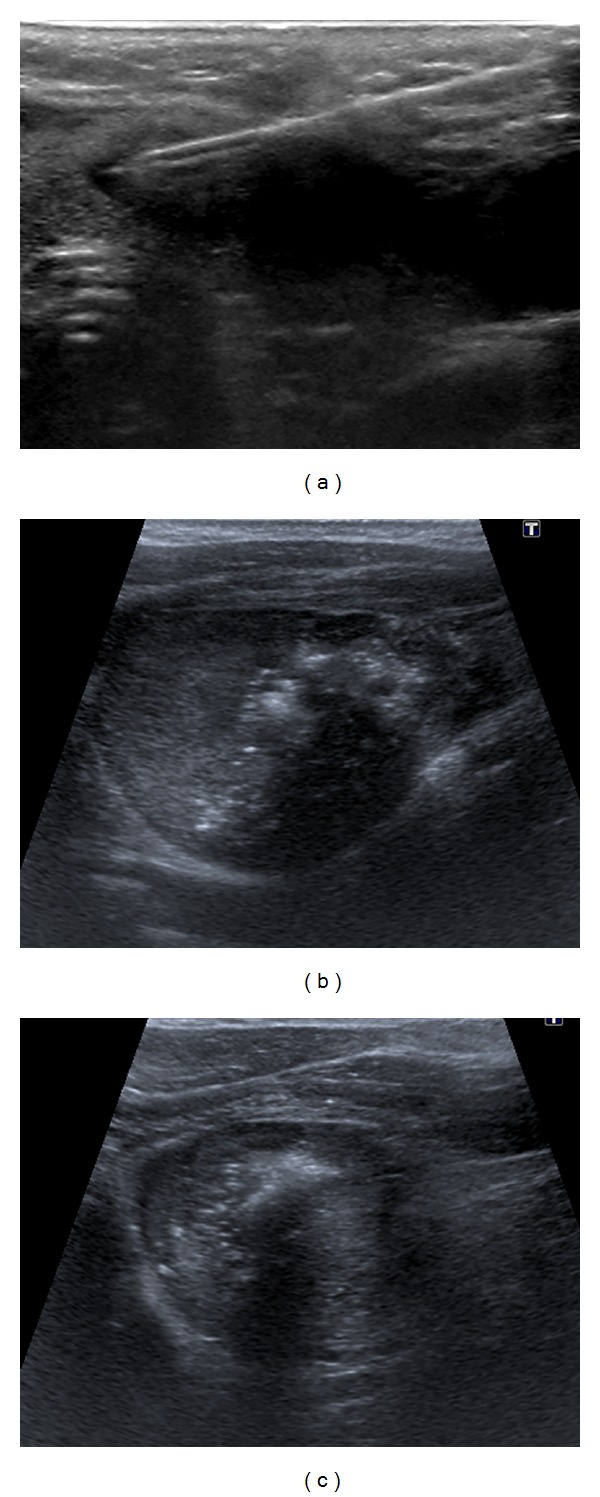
(a) Transverse US image shows the transisthmic approach. The probe, whose active part is placed in the nodule through its isthmus, is inserted from the medial to the lateral part of the nodule and is visible along its longitudinal axis. (b-c) Transverse US images show the moving-shot technique. RFA is performed unit by unit, aiming at ablating all the subunits of the nodule, which turn into microbubbles (hyperechoic areas with posterior barrage of the ultrasound beam). Initially, (a) the probe tip is positioned in the medial and deepest part of the nodule and subsequently (b-c) in its most superficial and lateral parts.
